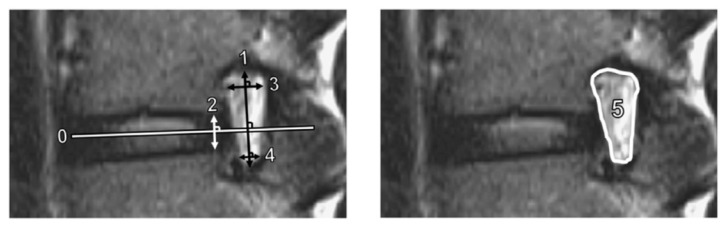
Measurements of magnetic resonance imaging parameters. Line 1 is the foraminal height; Line 2, disc height; Line 3, superior foraminal width; Line 4, inferior foraminal width; and Area 5, foraminal area. The anterior–posterior axis of the intervertebral disc (Line 0) was identified first. Lines 1 and 2 were constructed perpendicular to Line 0. Lines 3 and 4 were constructed parallel to Line 0.
